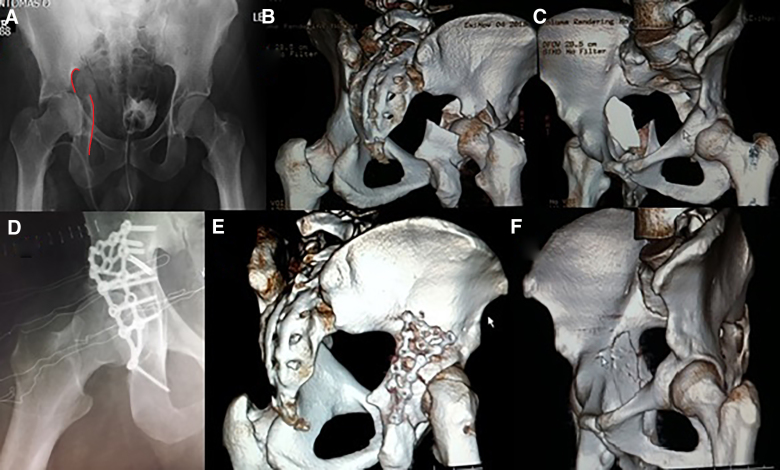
(A) Preoperative x-ray of a right posterior column and posterior wall fracture (fracture lines in red); (B, C) preoperative computed tomography images of the fracture; (D) postoperative x-ray of the Paseos plate application; (E, F) postoperative computed tomography images of the fracture.
